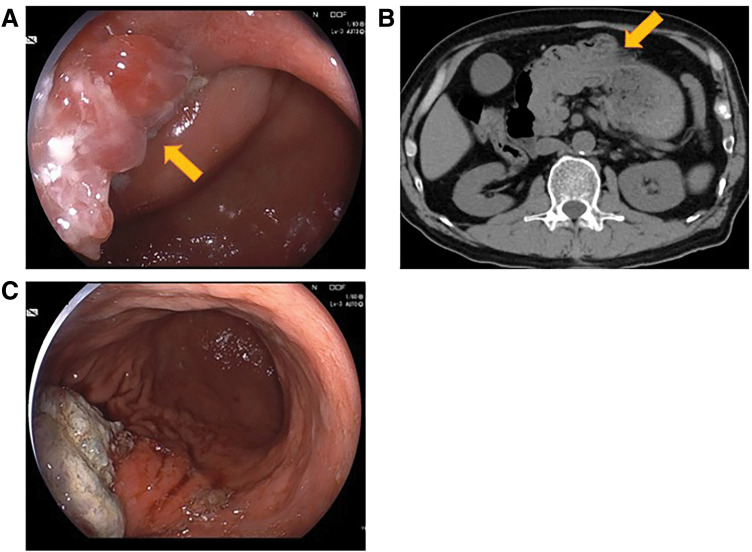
Findings during re-examination after the onset of epigastric pain. (A) Upper gastrointestinal endoscopy showed the tumor was shifted toward the duodenum. No additional findings, such as gastrointestinal bleeding, were observed. (B) Plain CT scan showed that the tumor had shifted toward the duodenum. (C) Upper gastrointestinal endoscopy after the tumor was repositioned into the stomach. The yellow arrow indicates the tumor prolapsing through the pyloric ring.
